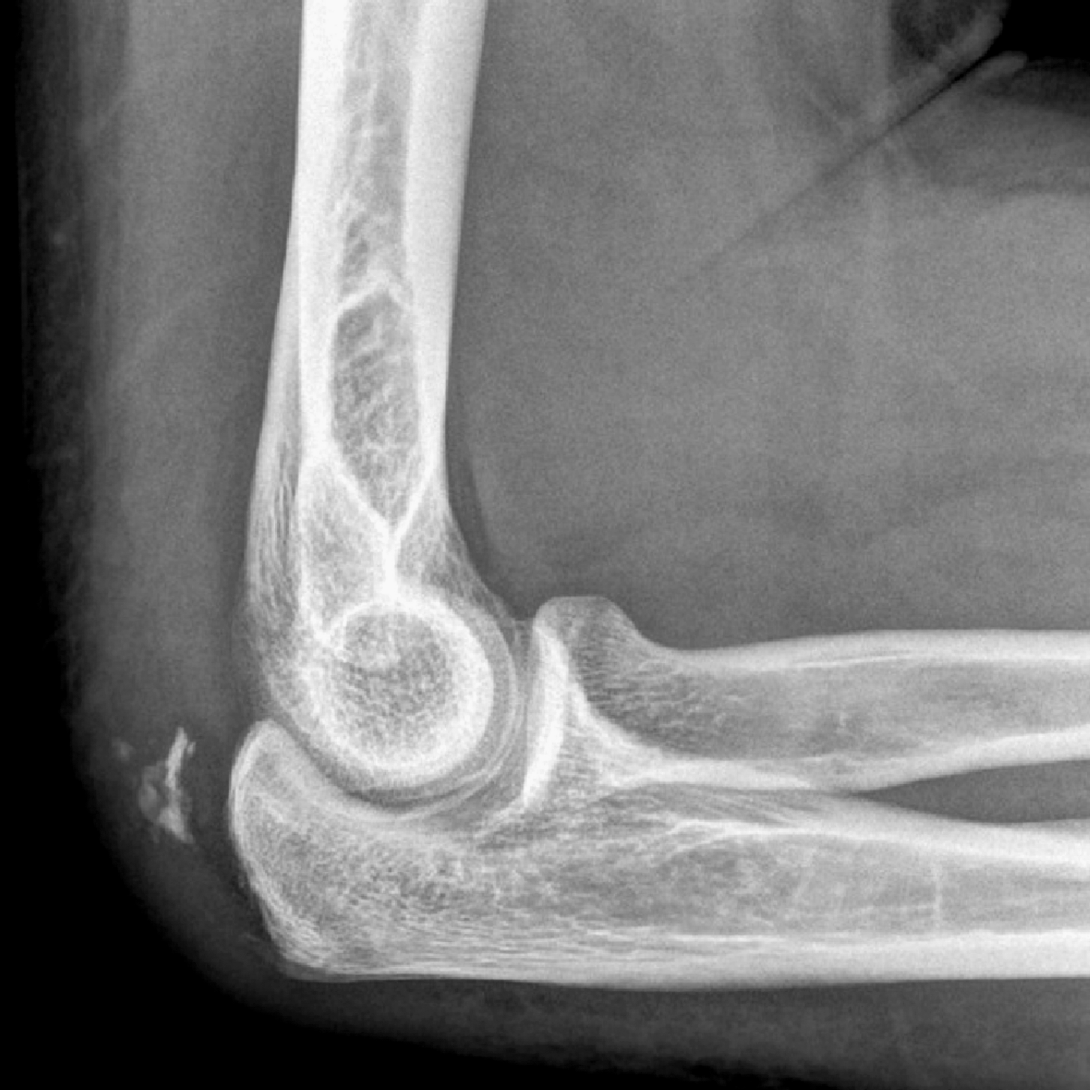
"Flake of bone"-typical sign on lateral X-ray image for triceps avulsion injuries Adapted/reproduced from Geyer et al. [37], with permission
